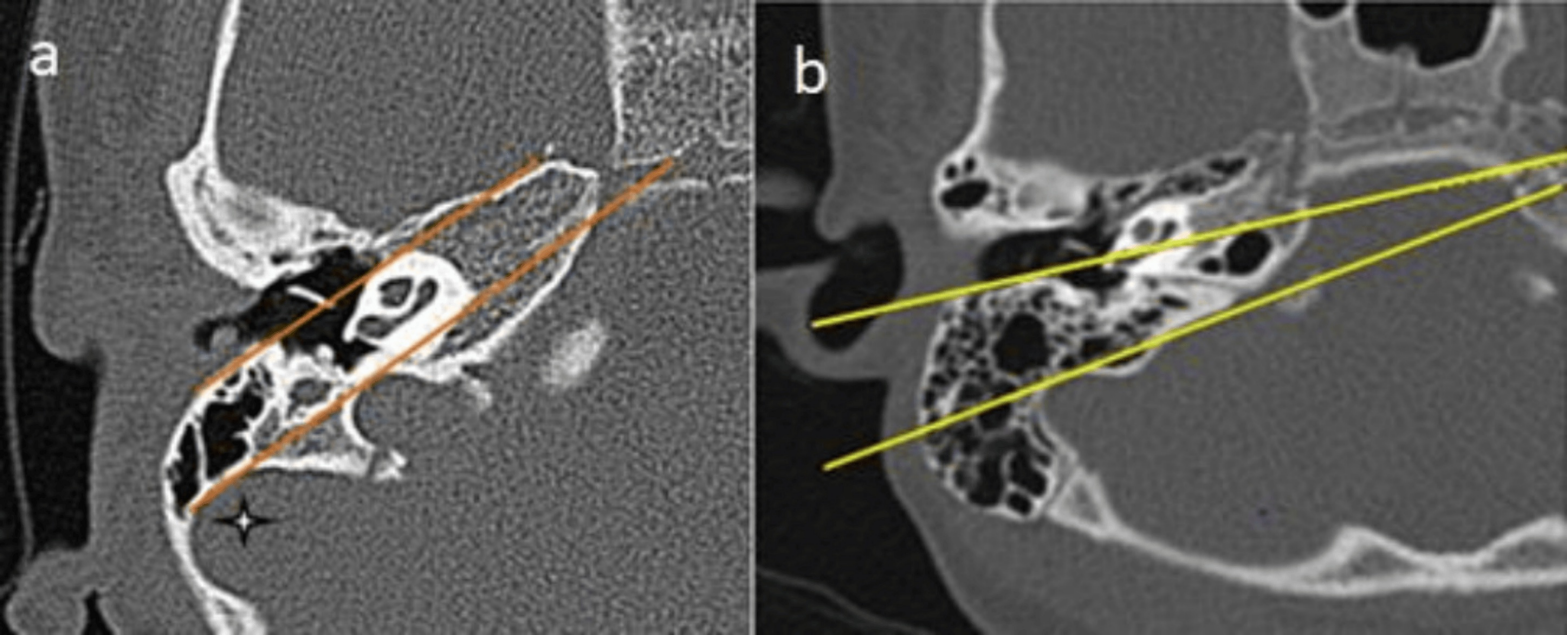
(a,b) Cochlear basal turn in relation to the posterior wall of the external auditory canal and anterior wall of the sigmoid sinus HRCT of the temporal bone showing (a) the cochlear basal turn in between the lines along the posterior wall of the external auditory canal and along the anterior wall of the sigmoid sinus (star) and (b) the cochlear basal turn which is lying outside both lines. HRCT: high-resolution CT
